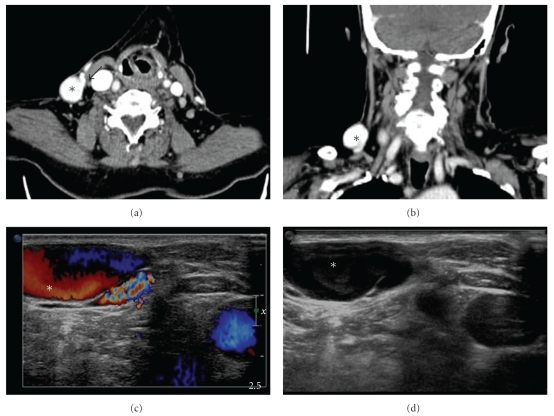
Axial (a) and (b) coronal Multidetector Computed Tomography (MDCT) images following intravenous contrast administration and color Doppler (c) and gray scale (d) ultrasound images of an external jugular vein aneurysm. Ultrasound and MDCT showed the presence of a cystic mass (asterisks) communicating with a short neck (arrow) with the external jugular vein. The lesion presented with venous flow on colour Doppler US examination and intense contrast enhancement on MDCT.
